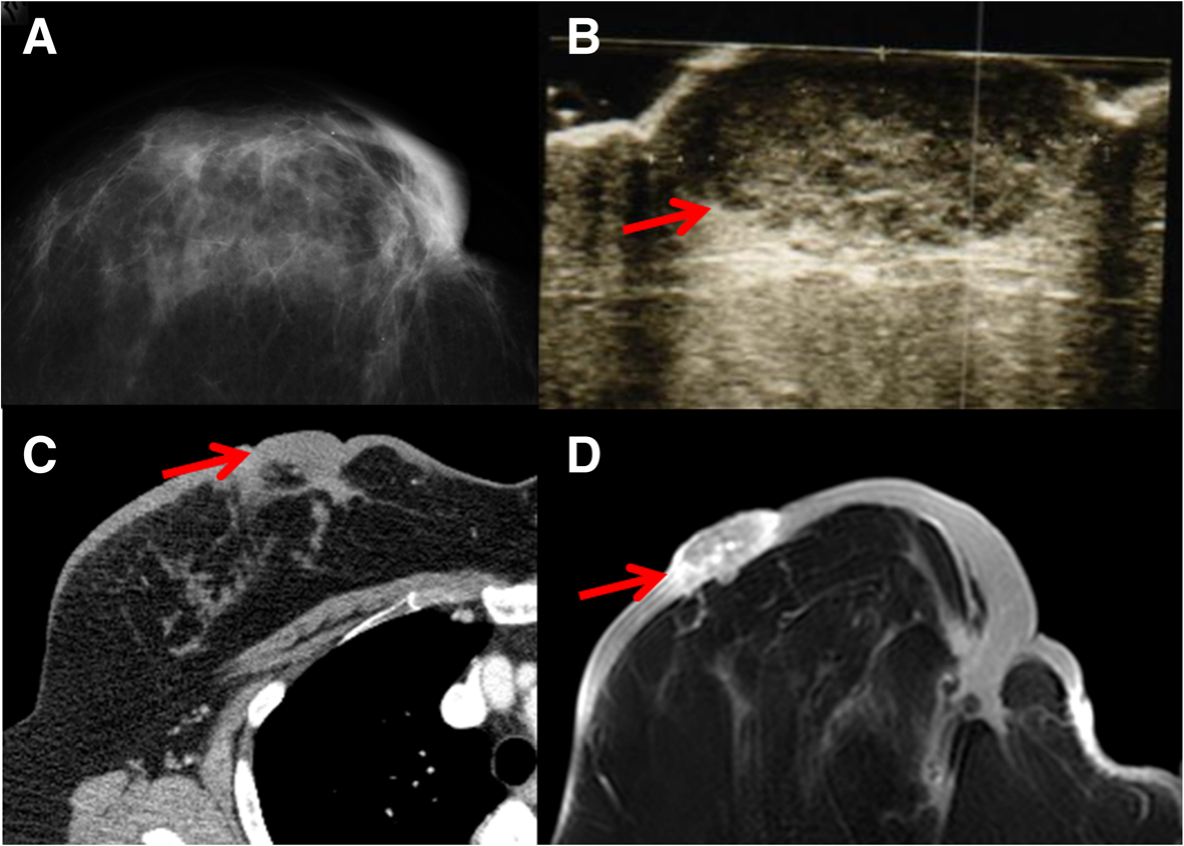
Imaging findings (case 1). a Mammography. b Breast ultrasound. c Computed tomography. d Magnetic resonance imaging. Arrow: tumor
